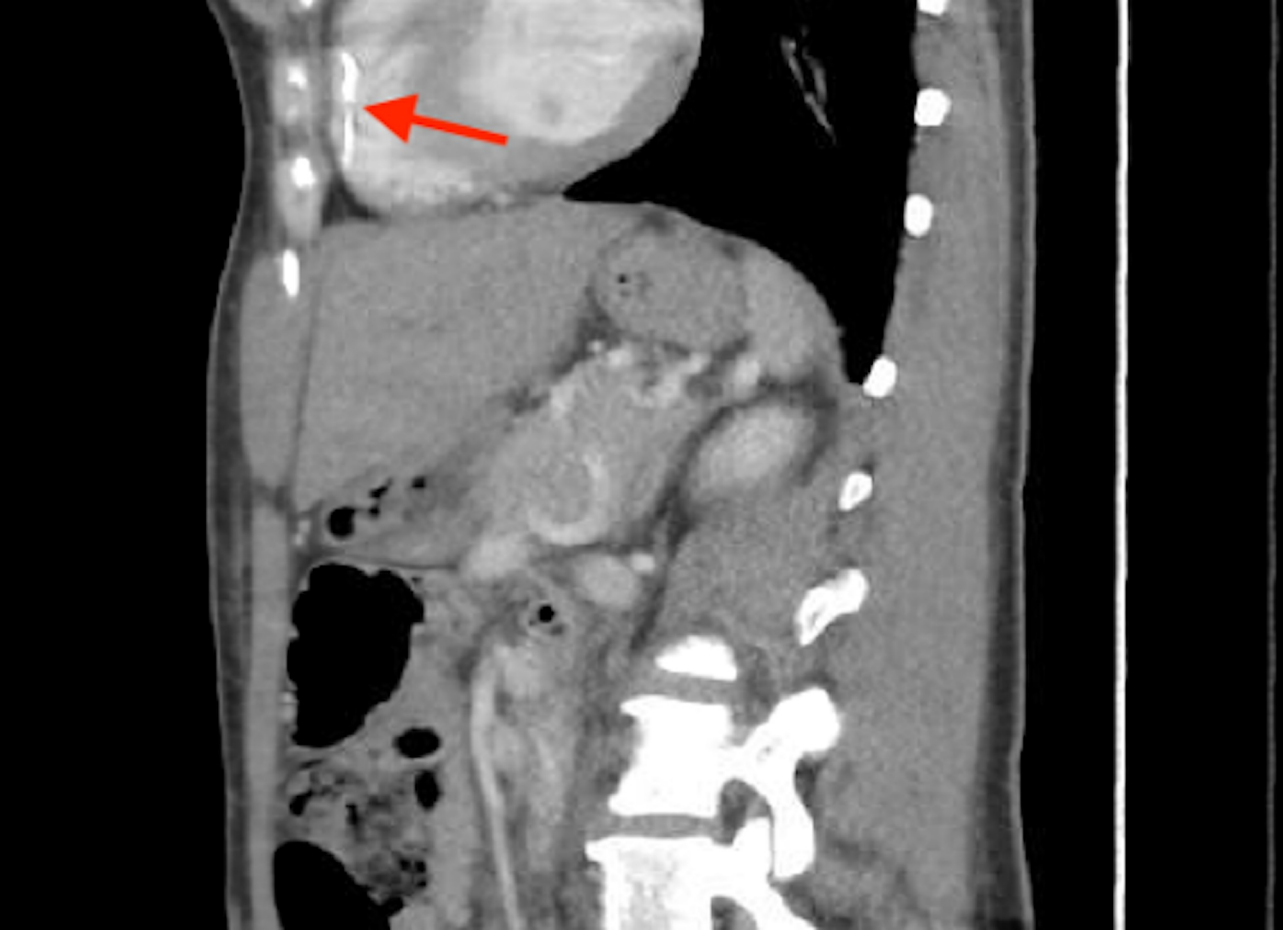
CT of the chest and abdomen (sagittal section) showing the migrated inferior vena cava filter strut (red arrow).
